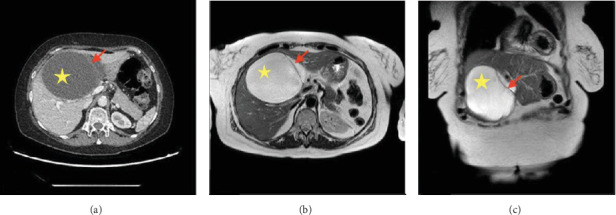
(A) Computed tomography image in axial projection shows a multilocular cystic tumor 62 mm × 53mm covering hepatic segments 4 and 5, where an internal septum is diffusely visible. (B) T2 sequence magnetic resonance in axial projection and (C) T2 sequence magnetic resonance in coronal projection shows the septum mentioned above is seen more clearly.
